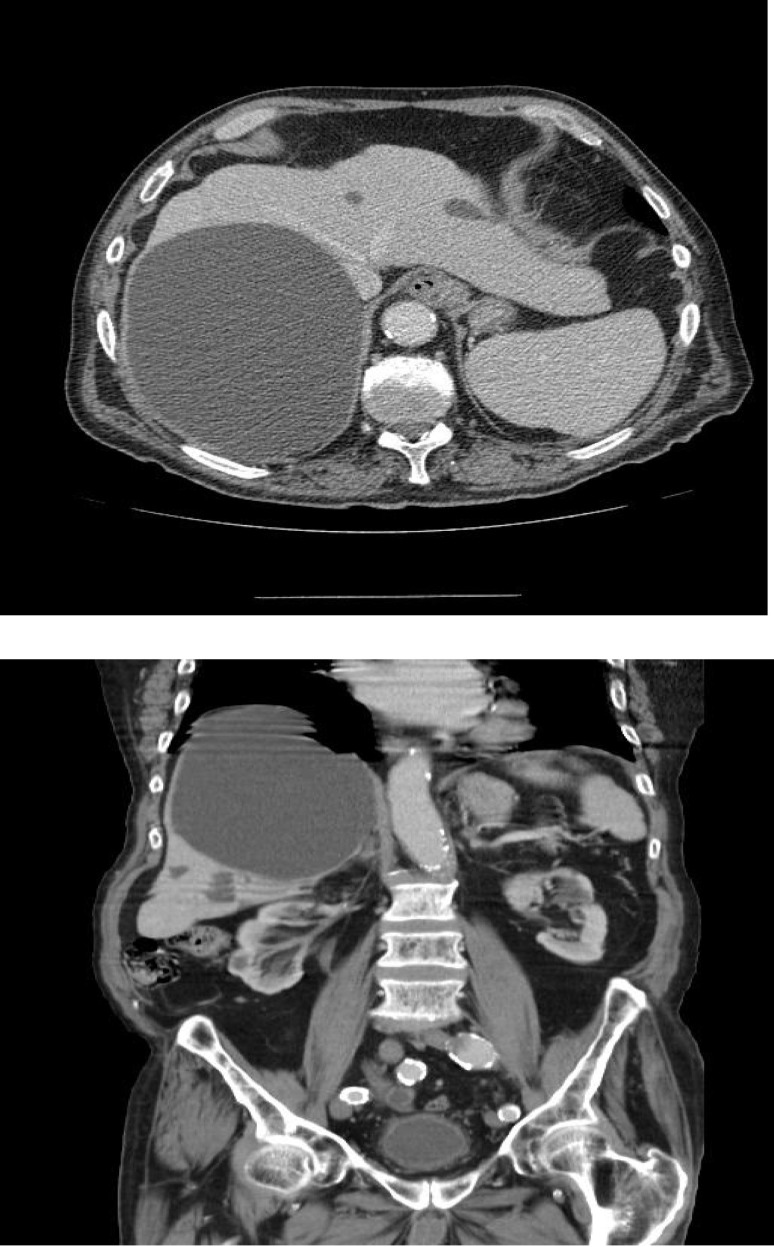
Images of CT in our research - simple cyst in the right lobe of the liver
